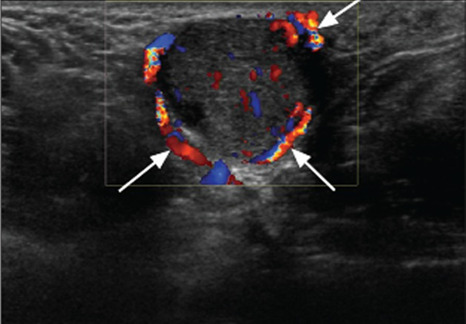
White arrows indicate point-of-care-ultrasound color Doppler image of axillary abscess showing minimal central vascularity, increased peripheral vascularity, posterior acoustic enhancement, and round hypoechoic central contents of abscess.^14^
